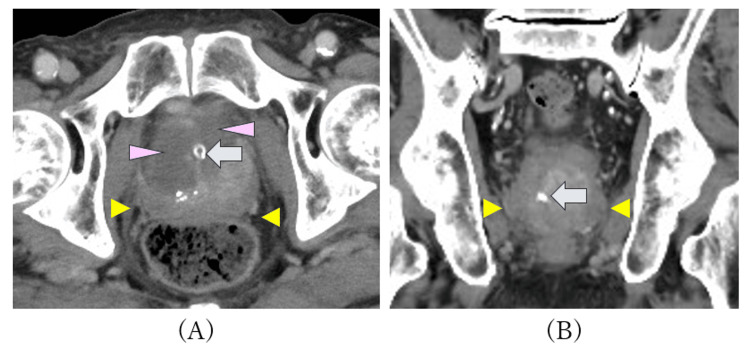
Axial (A) and coronal image (B) contrast-enhanced CT on postoperative day 6 Enhancement of the prostate and haziness of the periprostatic fat tissue (yellow arrowhead). Low-density area associated with the effect of WAVE procedure (purple arrowhead). Urethral catheter (blue arrow). CT: computed tomography, WAVE: water vapor energy therapy
